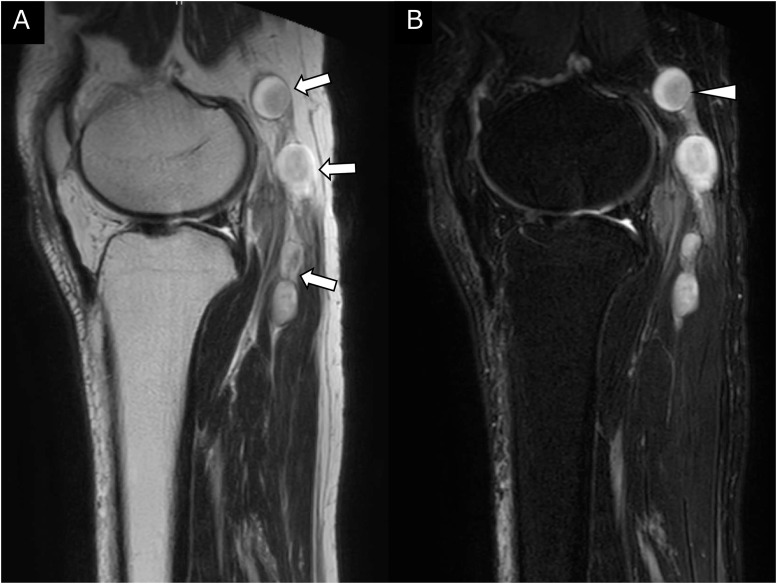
MRI of the left popliteal fossa. (A, B) Sagittal T2WI and fat-suppressed T2WI showed that a multinodular bead-like structure (arrows) was depicted along the tibial and peroneal nerve pathway. The nodules showed heterogenous high signal intensity, and eccentric relatively low-intensity areas (arrowheads) were also observed.
